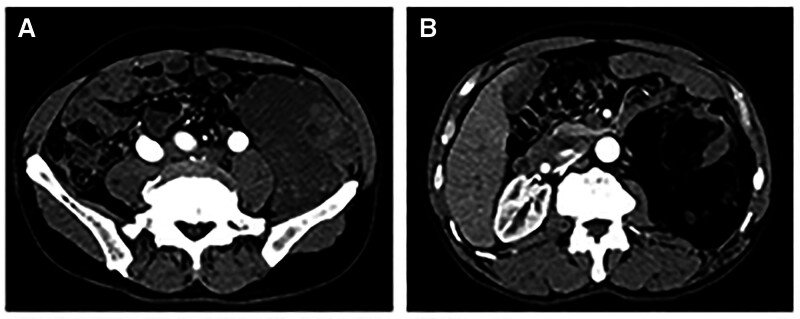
CT scans: solid mass shadow with uneven density in the left posterior abdominal cavity (A); mixed-signal shadow with predominantly fat components (B). CT = computed tomography.
